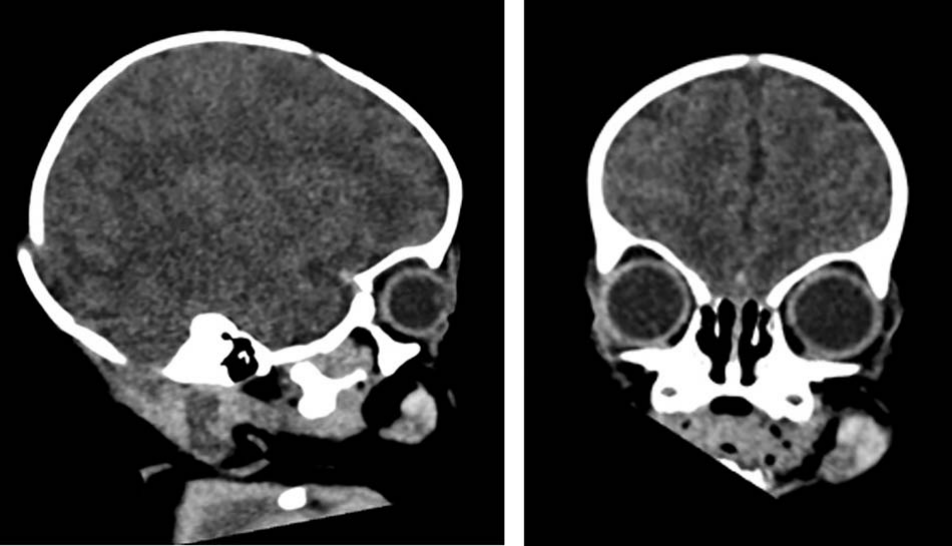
CT scan image (sagittal plane—left, coronal plane—right), showing 1–1.2-cm subcutaneous hyperdensity left buccal area with haematoma due to a possible pre-existing vascular malformation and no intracranial haemorrhages.
